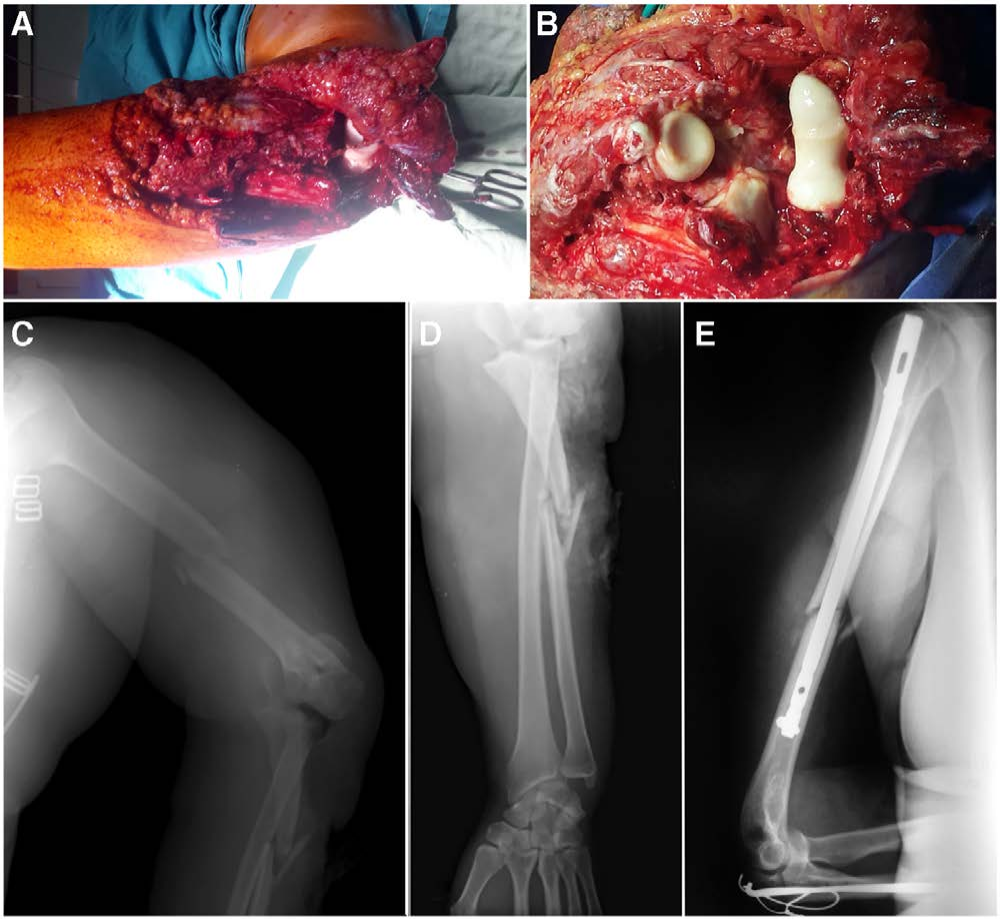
(A) Clinical appearance of the posttraumatic elbow wound. (B) Intra-articular elbow wound detail with direct visualization of elbow dislocation and olecranon fracture. (C) Initial X-ray (antero-posterior view) of the arm – displaced middle third, oblique, humeral shaft fracture, olecranon fracture and postero-medial elbow dislocation. (D) Initial X-ray (antero-posterior view) of the forearm – displaced proximal third, complex ulnar fracture, also reveals the medial elbow dislocation. (E) Postoperative X-ray (lateral view) of the arm - humeral shaft fracture reduction and fixation with a locked intramedullary nail, reduction of the olecranon and proximal third ulnar shaft fractures and fixation with a tension band, reduction of the elbow dislocation.
